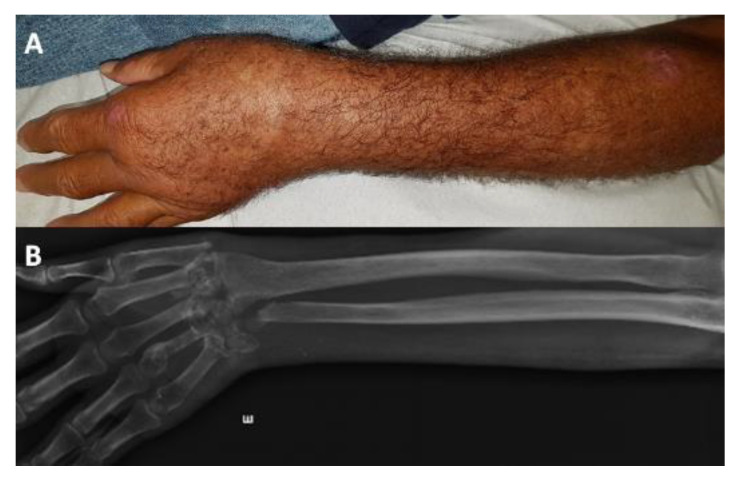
Disseminated sporotrichosis (cutaneous, tenosynovitis associated, and osteoarticular) in a 76-year-old man. (A) Scars on the skin of the left forearm along with swelling of the wrist. (B) Radiography showing extensive morphostructural alterations of the carpal bones, with loss of their individualization and wrist joint relationships in addition to deformity of the distal end of the 4th metacarpal and bone destruction of the distal epiphysis of the ulna.
